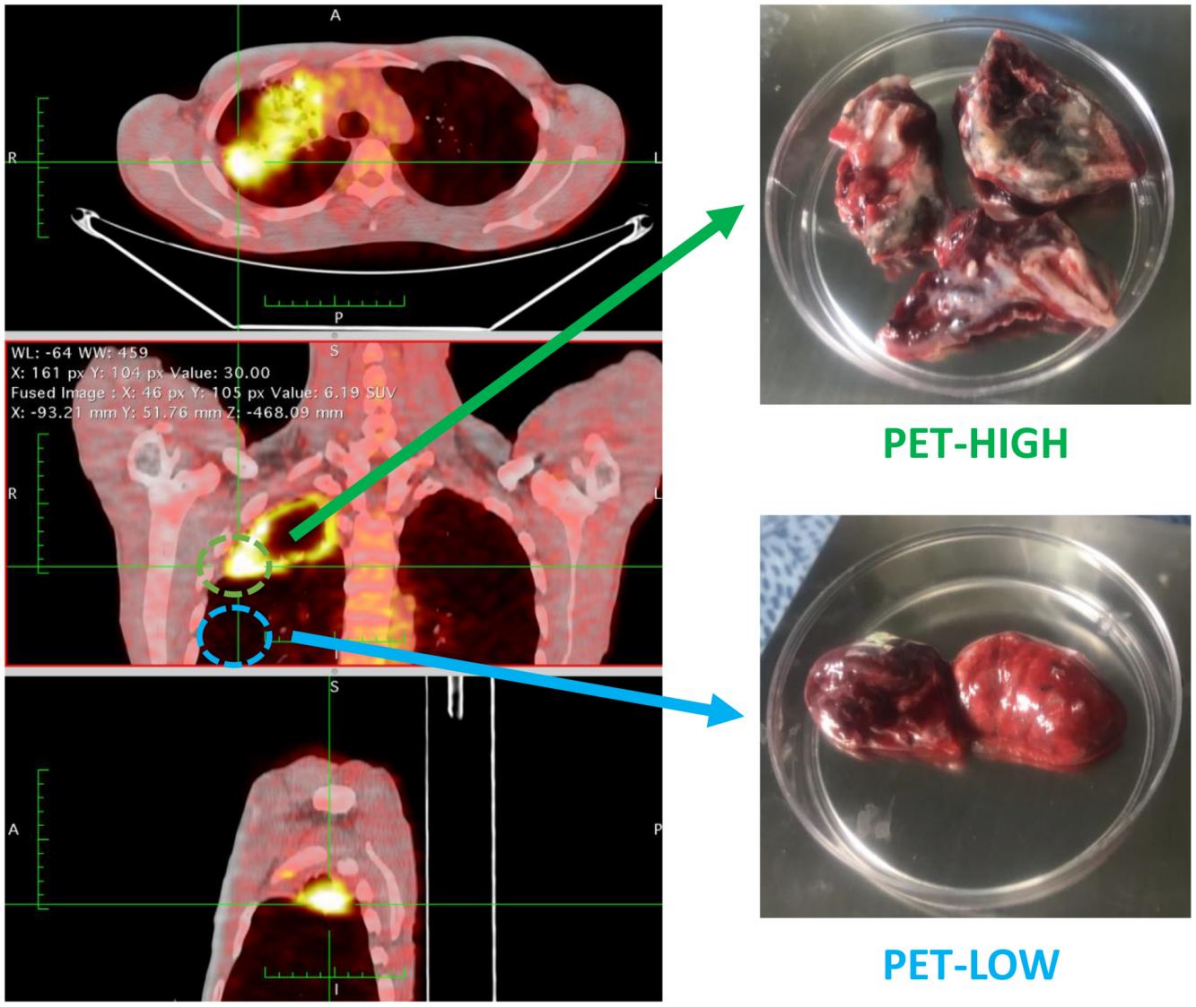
Lung tissue samples with high metabolic activity (PET-high) and low metabolic activity (PET-low) demonstrated by fluorine-18-fluorodeoxyglucose positron emission tomography combined with computed tomography (FDG-PET/CT) were collected for RNA-sequencing.
